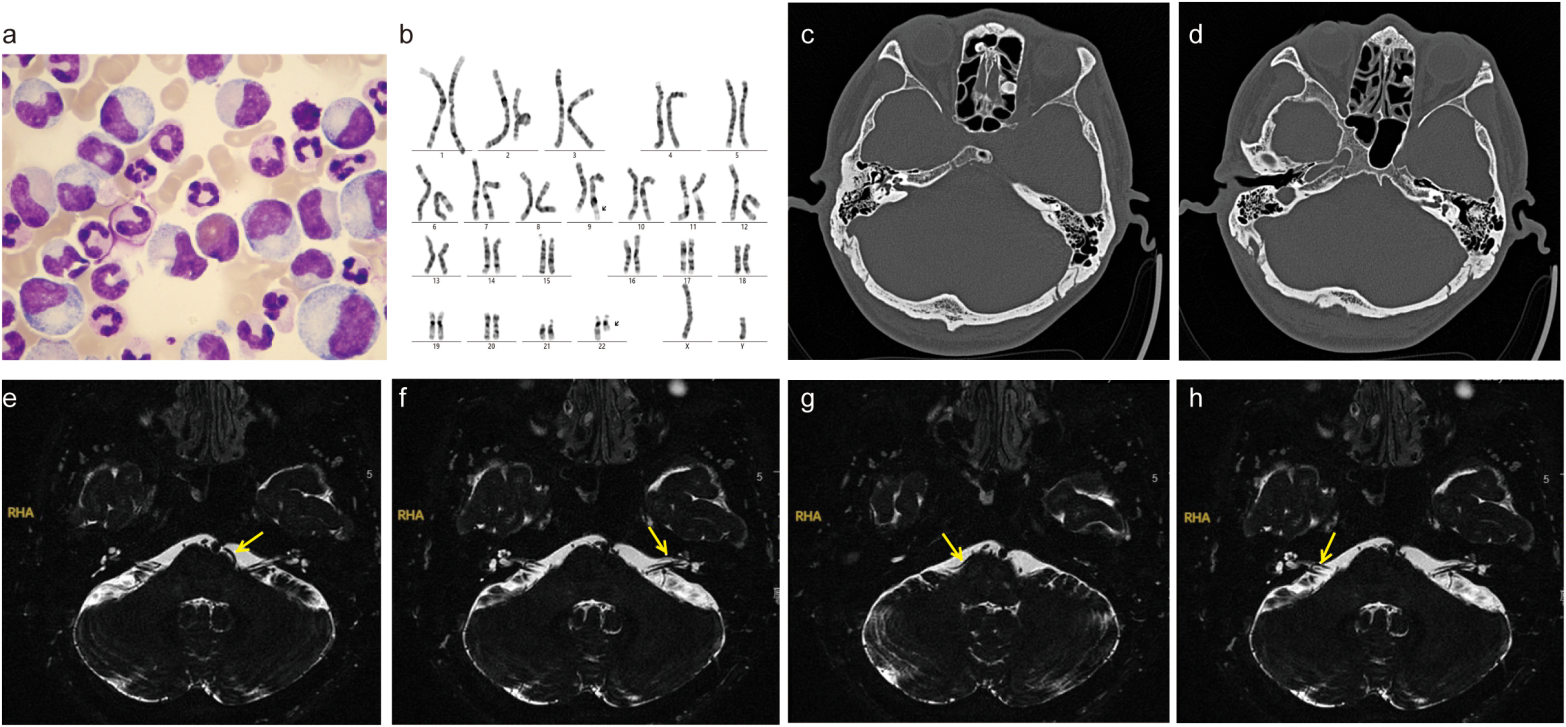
(a) A bone marrow cytomorphology image showing the extremely active proliferation of the granulocytic lineage (granulocytic lineage = 96%, erythrocytic lineage = 2%). (b) Chromosome karyotyping: 46, XY, t (9,22) (q34; q11). (c, d) Temporal bone computed tomography (CT) images showed no significant bilateral cochlear ossification. (e, f) Internal auditory canal magnetic resonance imaging (MRI) examination results; the yellow arrow indicates that the left anterior inferior cerebellar artery (AICA) was type IIB. (g, h) Internal auditory canal MRI examination results; the yellow arrow indicates that the right AICA was type IA.
